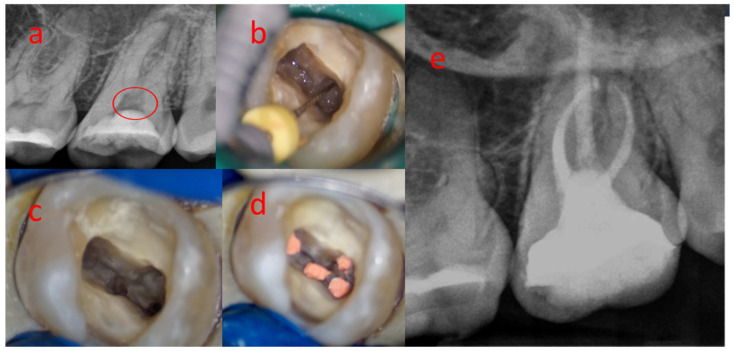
Removal of pulp mineralization followed by endodontic treatment: (a) Preoperative radiograph; (b) Identification of canal orifices; (c) The pulp chamber after the pulp stone removal; (d) Tooth after the endodontic treatment; (e) Postoperative radiograph.
